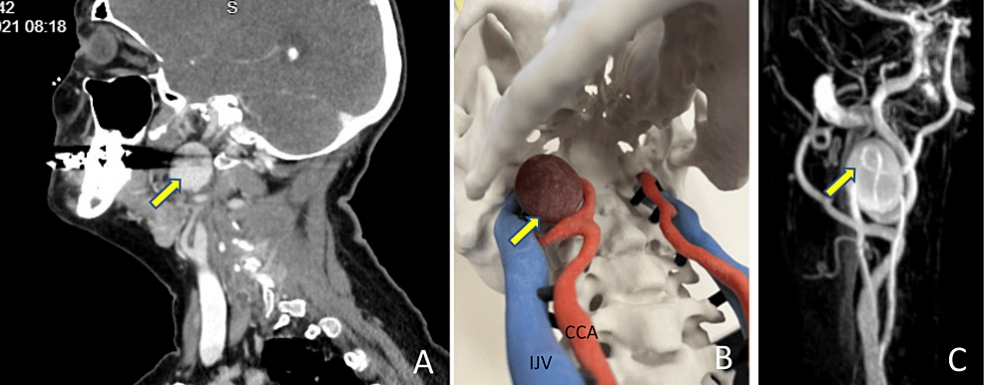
Preoperative imaging and 3D model (A) Sagittal view of CT scan demonstrating ECAA; (B) 3D model showing ECAA; (C) MRA showing aneurysm and its connection to ICA CCA: common carotid artery; IJV: internal jugular vein, yellow arrow: ECAA
